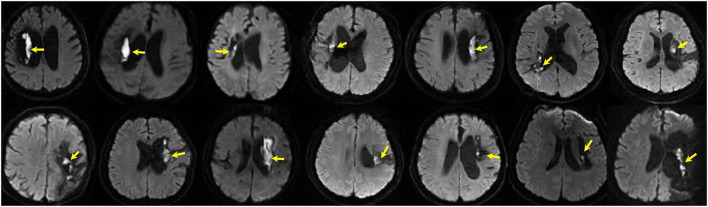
DWI hyperintensities (arrows) at previous hematoma in 14 patients of intracranial hemorrhagic with surgery (ICH–WS) at the chronic stage.
